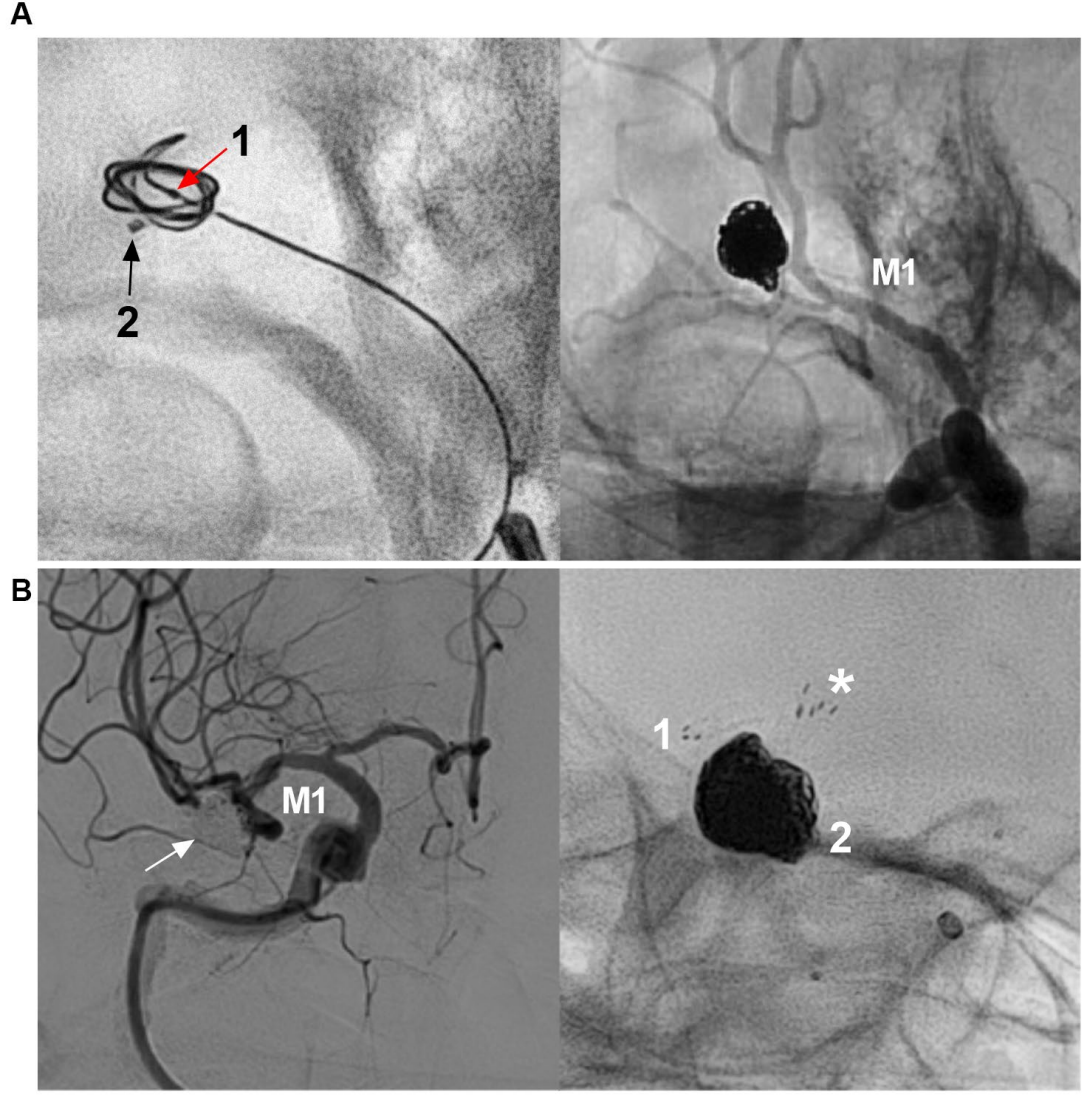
Traditional coiling EVT for MCA aneurysms. (A) Left panel: X-ray image showing two microcatheters into the aneurysm (arrows 1 and 2), microcatheter 1 was used for coiling first; Right panel: unsubtracted DSA showing that the aneurysm was coiled completely by the two microcraters alternately. (B) Left panel: DSA showing that the MCA bifurcation aneurysm (arrow) was completely coiled; Right panel: X-ray image showing that the “Y configuration” stents were used, asterisk indicated the proximal markers of the two stents. Numbers 1 and 2 indicate the distal markers of the two stents. CTA, computed tomography angiography; DSA, digital subtraction angiography; EVT, endovascular treatment, MCA, middle cerebellar artery; M1, M3, first and third segments of the MCA.
